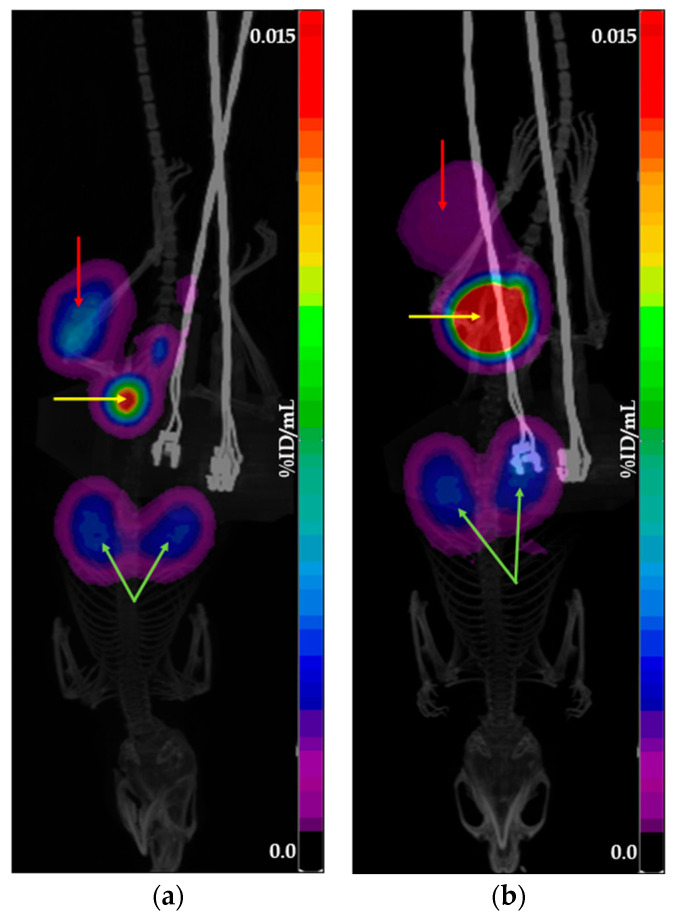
Example of PET/CT scans obtained using [^68^Ga]Ga-DOTATOC: (a) produced with cyclotron solid target isotope; (b) produced with generator-origin isotope. The images show the main places of tracer accumulation: the red arrow indicates the tumor, the yellow arrow indicates the bladder, and the green arrow indicates the kidneys.
